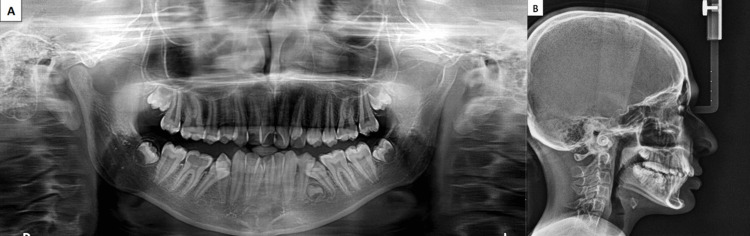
Pre-treatment radiographic records A. Preoperative orthopantomogram. B. Preoperative lateral cephalogram.
